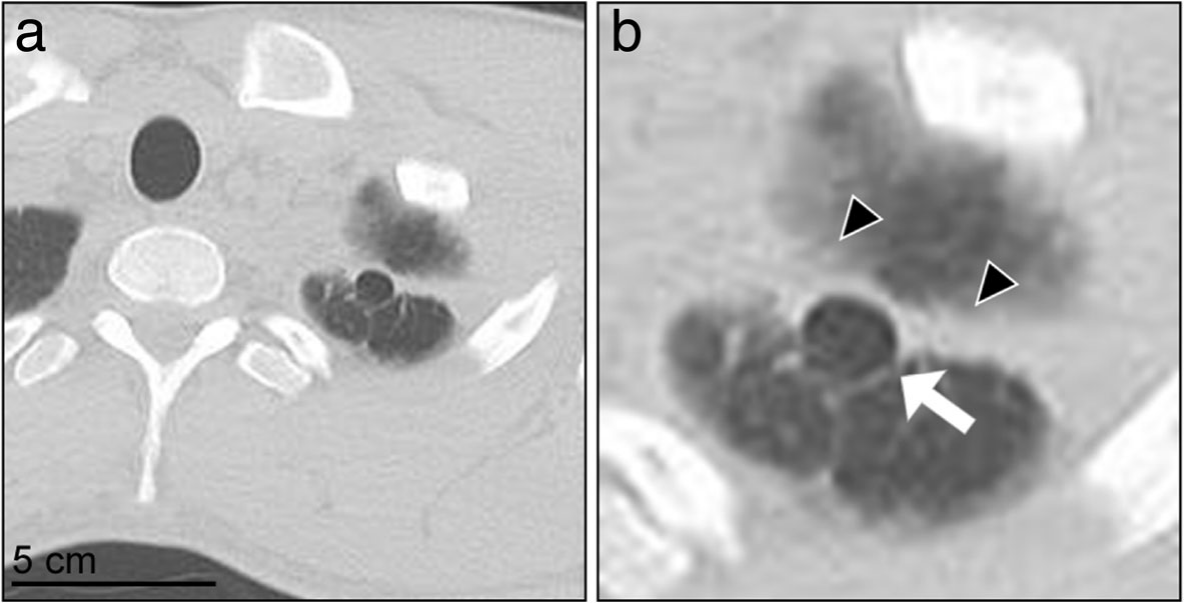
One-year chest computed tomography scan showing typical neogenetic bullae adjacent to the staple line. a Lower magnification of the left apex; b Higher magnification of the left apex. The white arrow indicates the neogenetic bullae, while the black arrowheads indicate the staple lines
